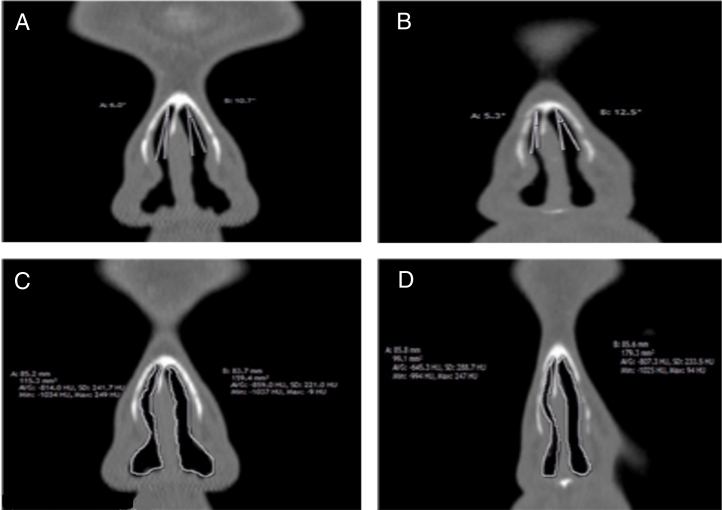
Internal nasal valve angle/area pre and postoperative by reformatted coronal CT-scans. A, angle pre-operative; B; angle post-operative; C, area pre-operative, D, area post-operative.
